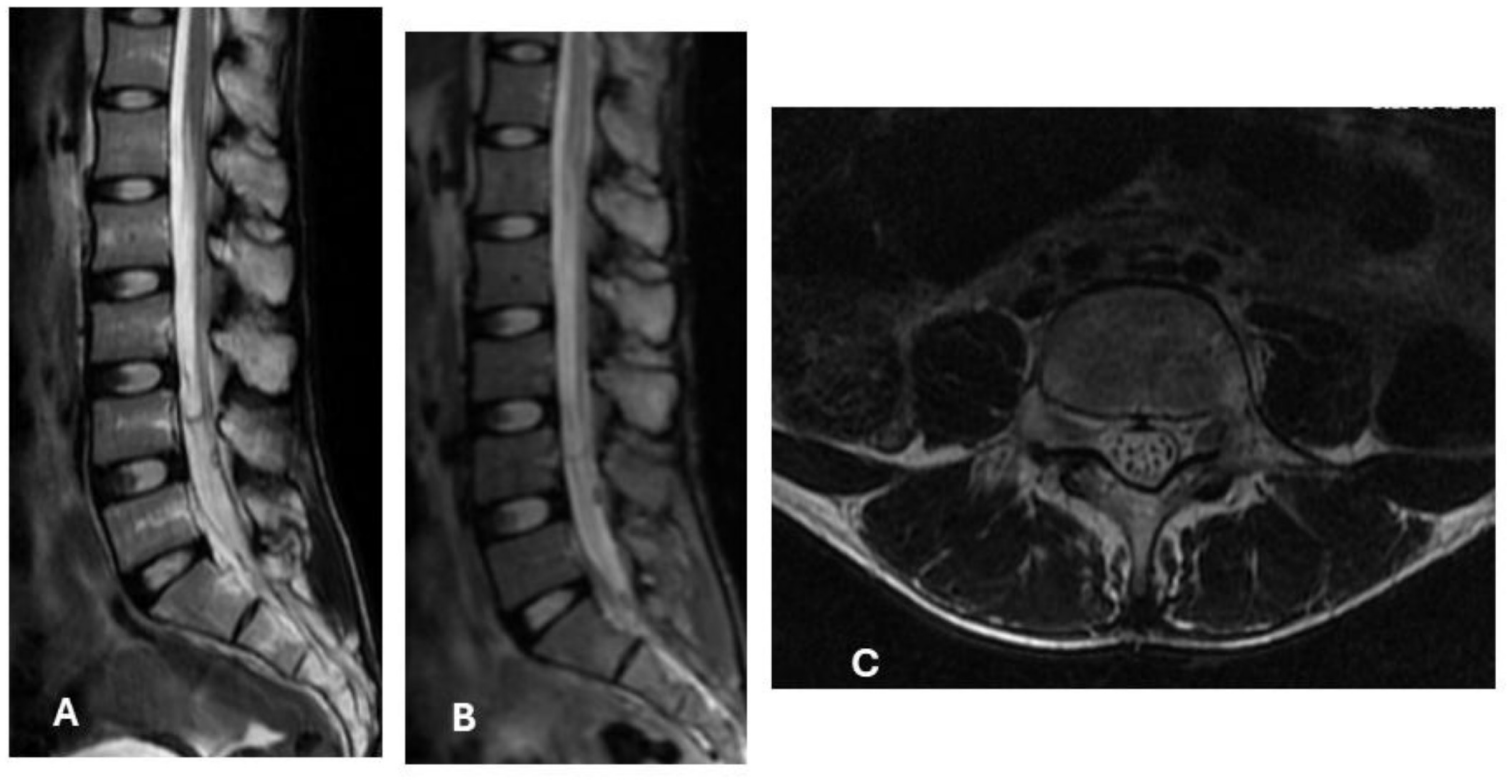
Sagittal section in T2 MRI showing intramedullary hyperintensity between Lumbar segments L1 to L4 (A), and enhancing mass at the conus medullaris with extensive spinal cord edema (B). Intramedullary nodular lesions exhibiting T2 hyperintensity, associated with mild spinal cord enlargement, findings suggestive of schistosomal granulomas (C).
